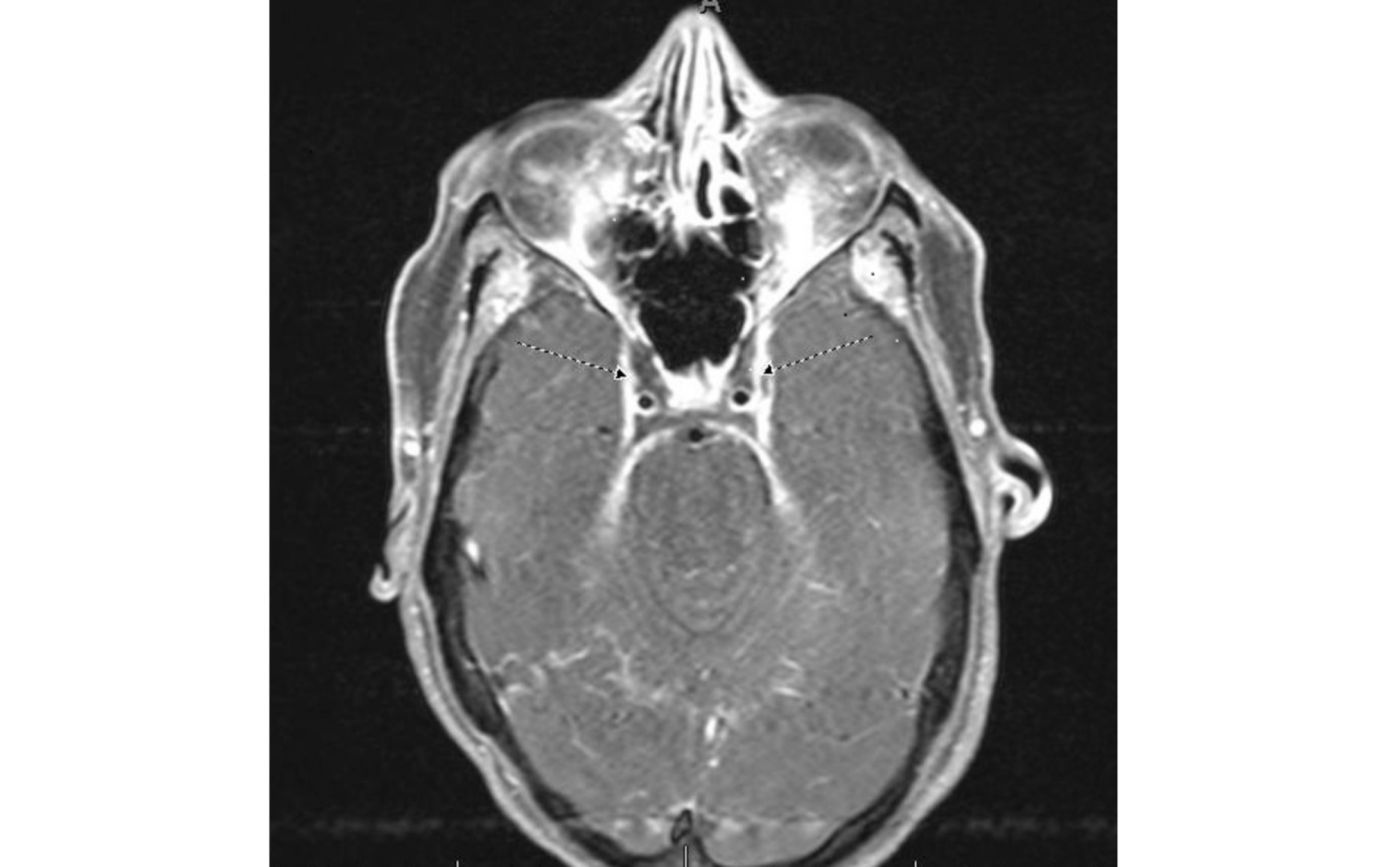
MRI of the brain/orbits, with and without contrast, showing bilateral cavernous sinus thrombosis (dotted arrows) with left retrobulbar enhancement and no evidence of sinusitis.
